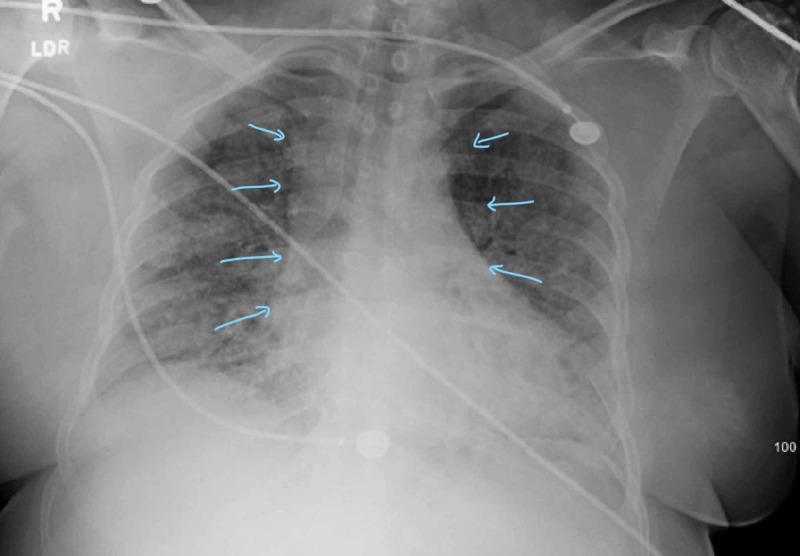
Chest X-ray showing bilateral ground glass opacities with worsening aeration suggestive of acute respiratory distress syndrome (ARDS) (arrows pointing)
